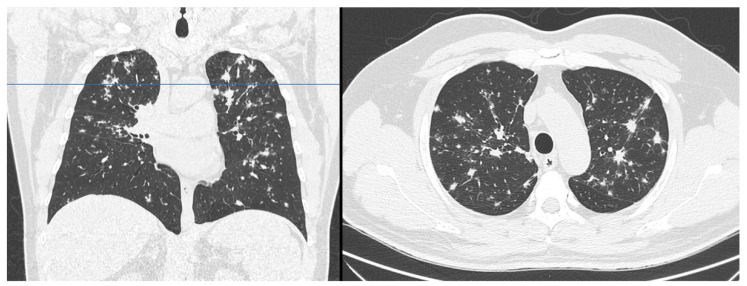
Chest computed tomography showed multiple peribronchovascular nodules, mainly located in the upper lobes.
